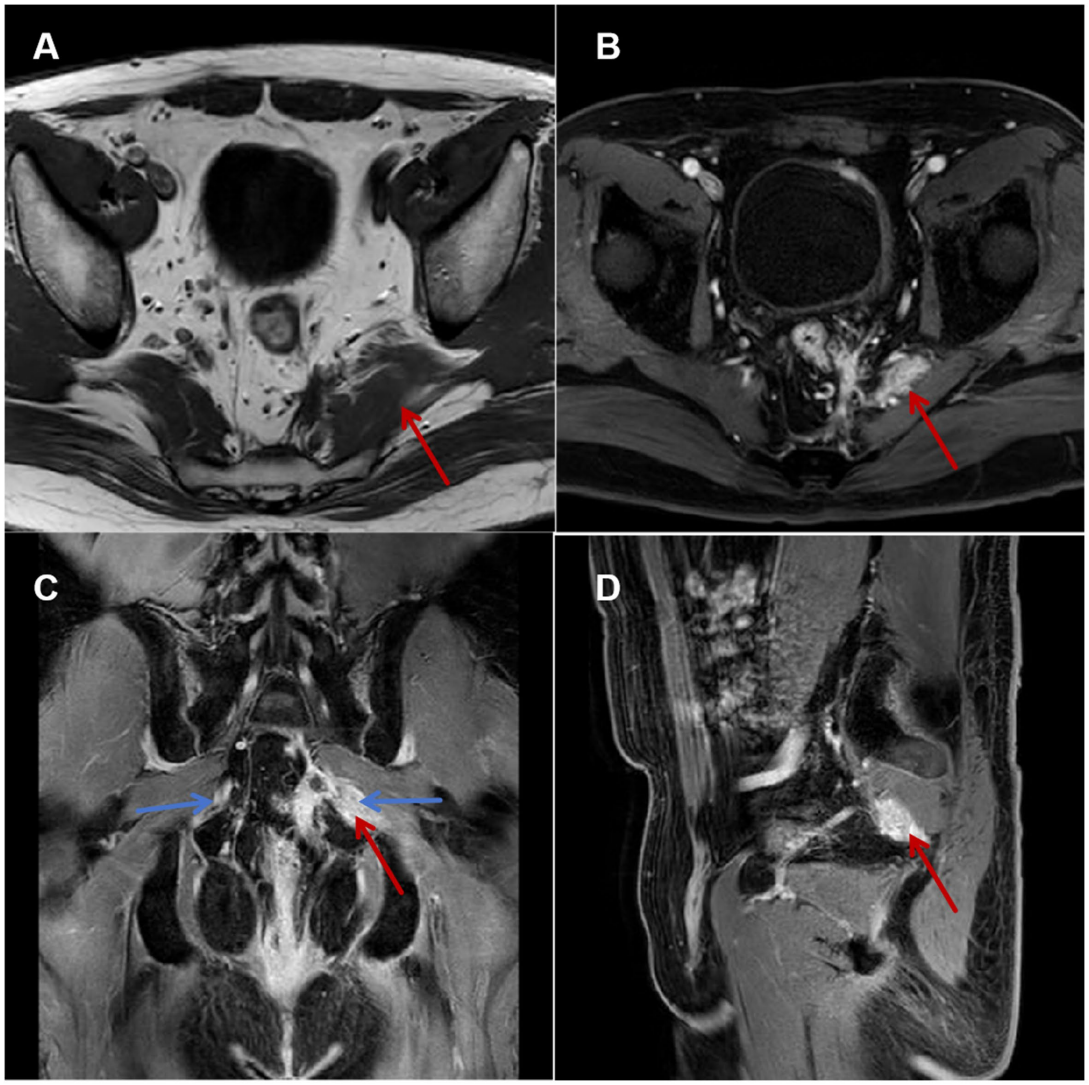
Pelvic floor MRI. (A) Axial T1-weighted image: Hypointense signal and thickening of the left piriformis muscle (black arrow). (B) Axial T2-weighted image: Heterogeneous hyperintense signal within the left piriformis muscle (black arrow), increased signal intensity in the surrounding fat layer. (C) Coronal contrast-enhanced-weighted image: Patchy enhancement surrounding the nerve course (blue arrow) in the left piriformis region, compared to the contralateral side. (D) Sagittal contrast-enhanced-weighted image.
